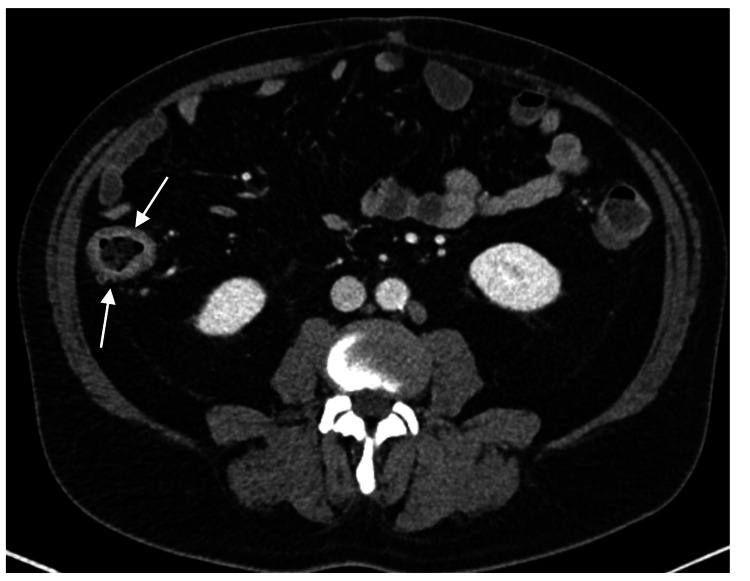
Abdominal CT scan, revealing ascending colon thickening walls (white arrows), suggestive of chronic colitis CT: computed tomography
